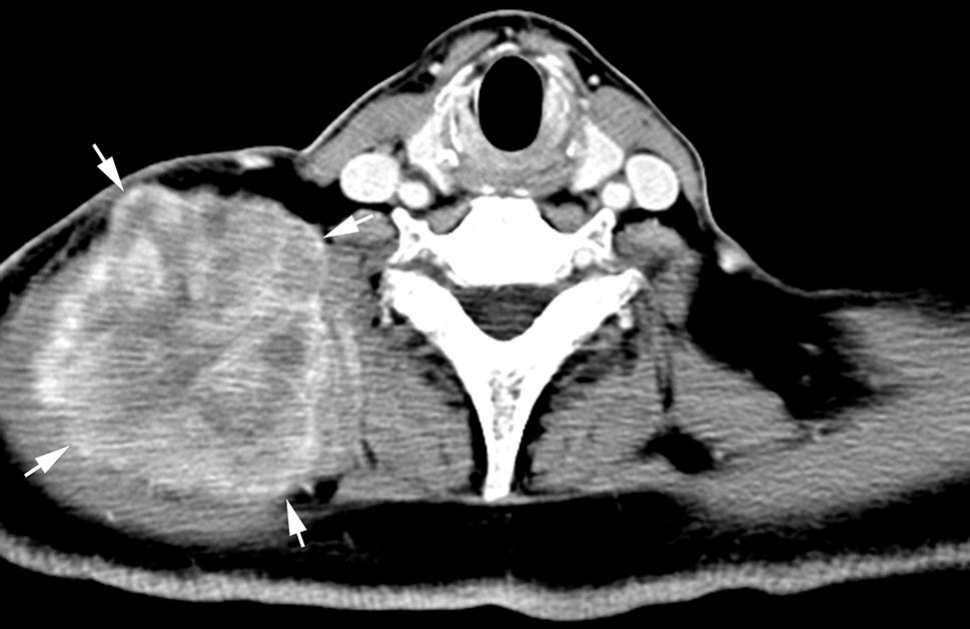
Radiation-induced fibrosarcoma 10 years after radiotherapy of a 50-year-old man. Axial contrast-enhanced CT image showed a large heterogeneously marked enhanced tumor with wide invasion into the muscles in the right lower neck (*arrows*)
